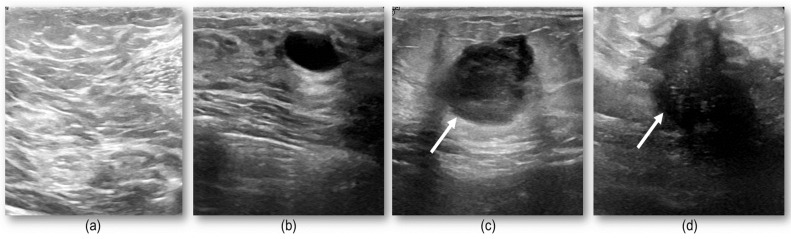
Four examples of ultrasound: (a) shows a normal case with no suspicious lesion, (b) depicts a benign nodule, while (c,d) show malignant tumors. These images show the challenge in discriminating different group of lesions with different textural complexities.
